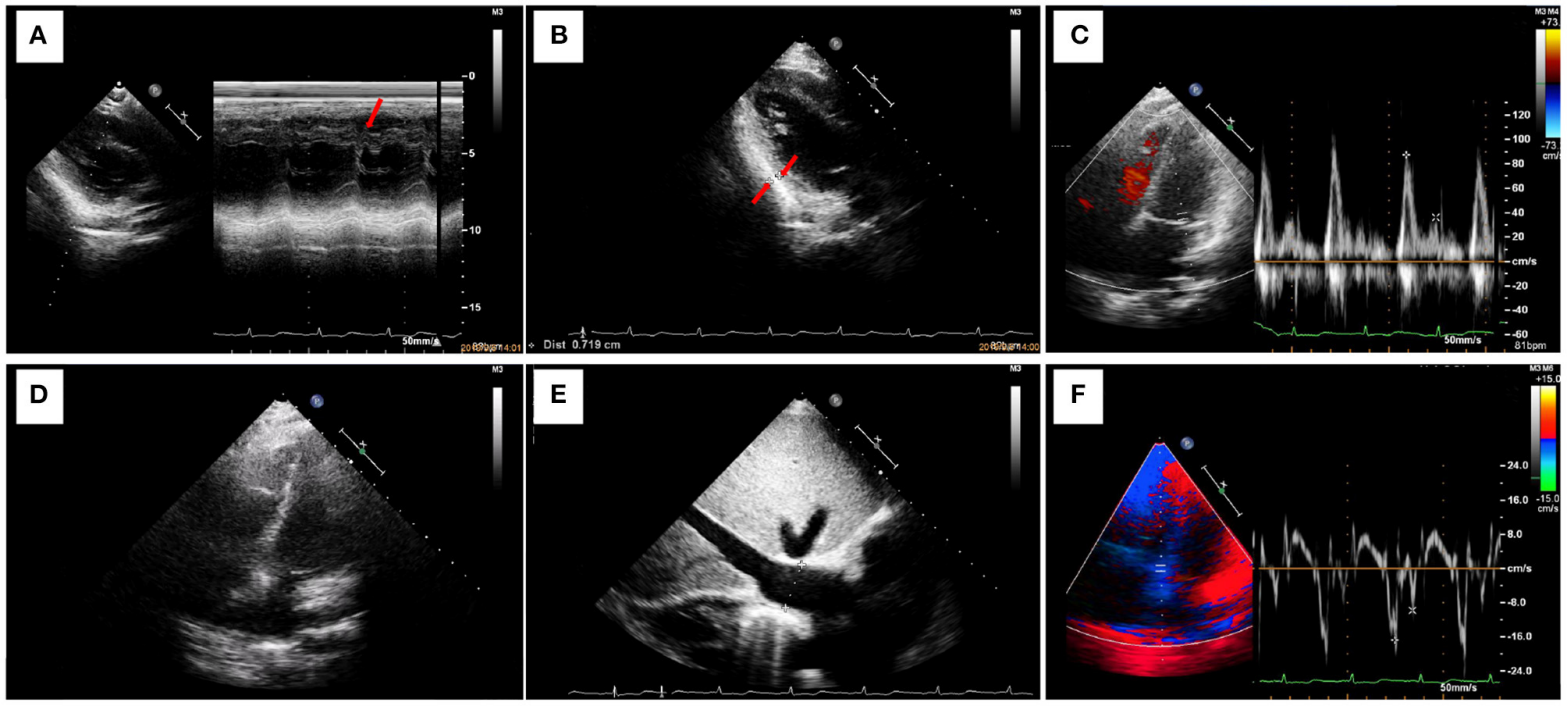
Ultrasonography shows decreased cardiac function underlying protein-losing enteropathy. (A) M-mode ultrasonography shows thickening of the posterior pericardium of the posterior wall of the left ventricle and signs of a septal notch. The left ventricular ejection fraction is 77%. (B) The posterior pericardium of the posterior wall of the left ventricle is thickened (thickness, 7 mm). (C) Pulse Doppler shows limited ventricular diastolic activity. The high peak E and low peak A result in an E/A of >2. (D) A four-chamber view of the heart shows marked thickening of the pericardium and atrial enlargement. (E) The inferior vena cava is greatly widened (width, 26 mm). (F) Myocardial relaxation is normal.
